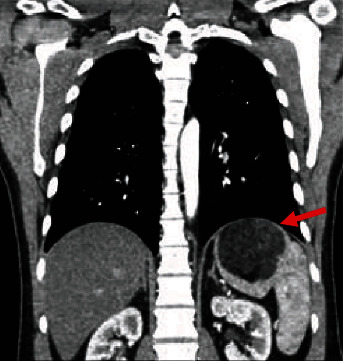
Contrast-enhanced computed tomography coronal image depicting the myelolipoma within the spleen (arrow).
